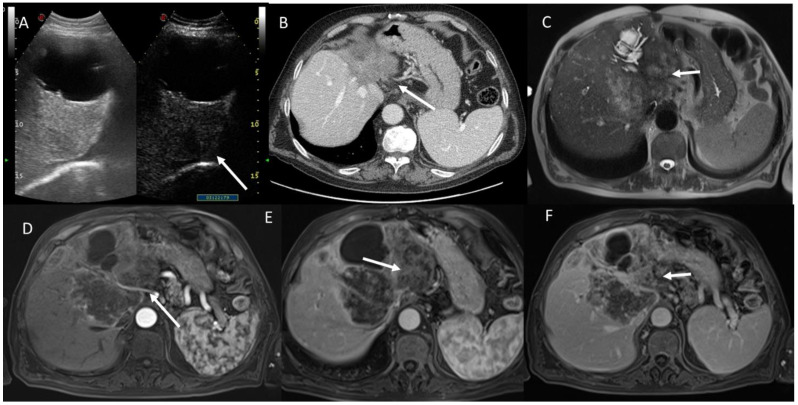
Imaging assessment of degenerated intraductal papillary neoplasms of the bile duct in a 78-year-old patient. (A) Contrast-enhanced ultrasound evaluation, with progressive contrast enhancement of invasive lesion component (white arrow). This pattern (white arrow) is also present in CT assessment ((B): portal phase) and in MRI contrast study (Volumetric interpolated breath-holding examination sequence in (D): arterial phase, (E): late arterial phase, and (F): portal phase). In T2-weigthed sequences (C), the invasive component shows inhomogeneous iso-hyperintense signal (white arrow).
